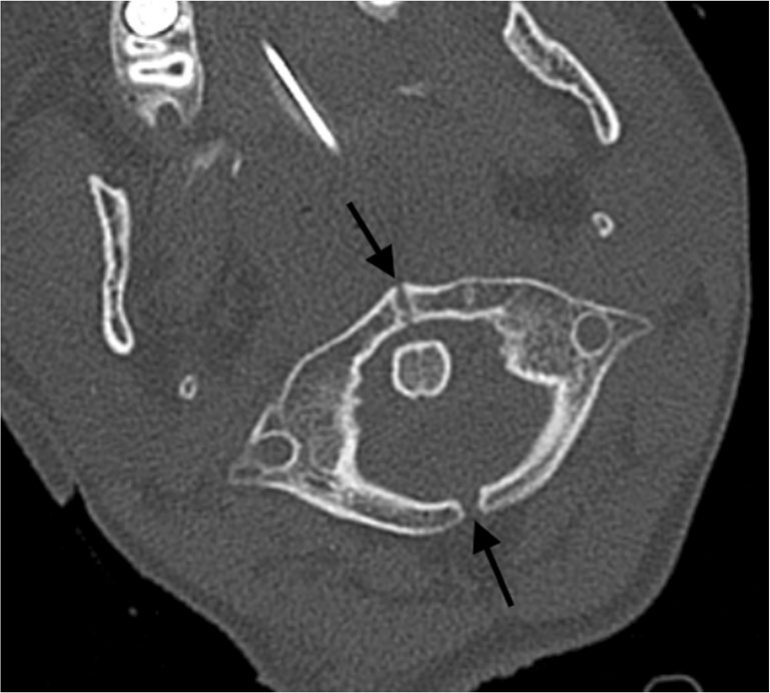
Split atlas: axial CT image through the atlas (C1 vertebra) of the craniocervical junction of a 9-year-old female struck by a car and sustaining severe chest injuries. The victim was intubated at the roadside. A developmental “split atlas” is present with anterior and posterior rachischisis defects present (*arrows*)—this is an important but rare mimic of fracture of the C1 vertebra. There were no acute fractures of the craniocervical junction in this patient
